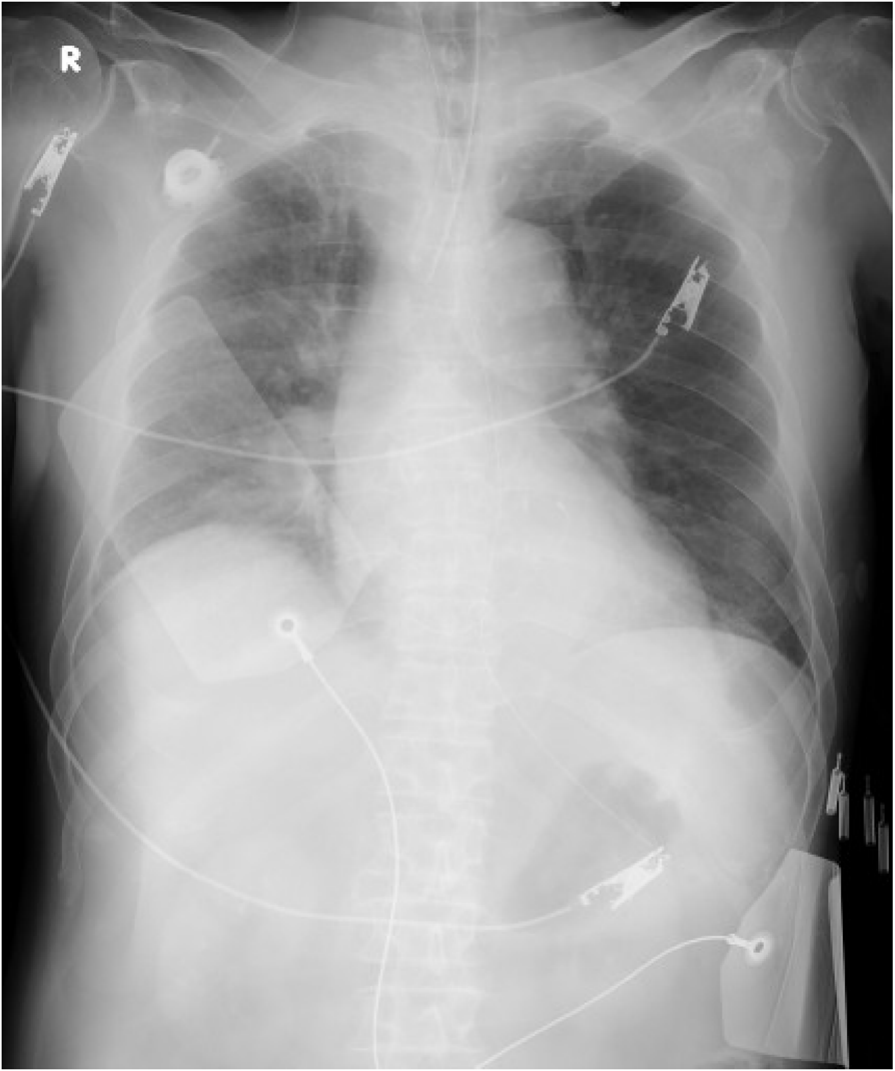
Chest X-ray, taken immediately after cardiopulmonary resuscitation, of a patient with heat stroke and cardiac arrest, indicated no apparent cardiopulmonary abnormalities
